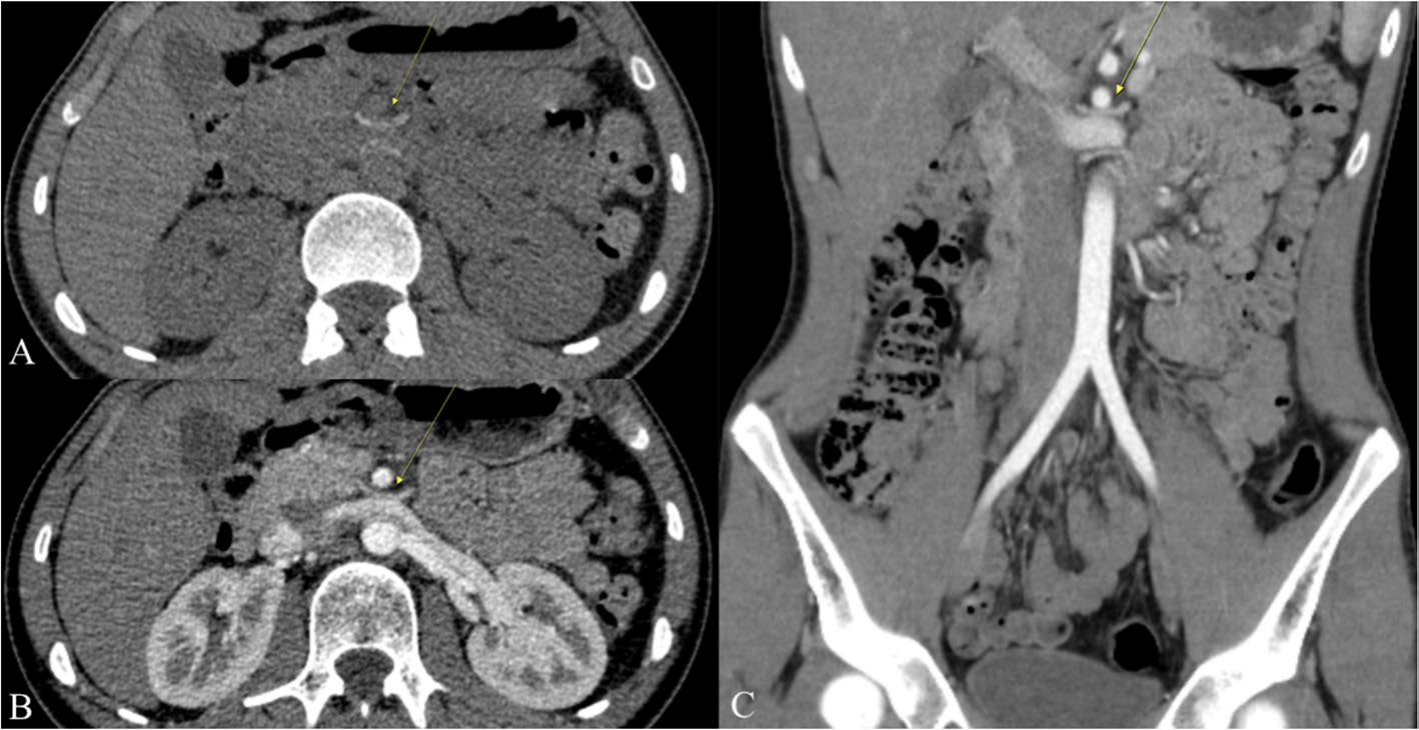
CT examination demonstrating patent 3D-printed PEEK extravascular stent due to Nutcracker syndrome (arrow): A non-contrast-enhanced, axial plane, B contrast-enhanced, axial plane, C contrast-enhanced, coronal plane
